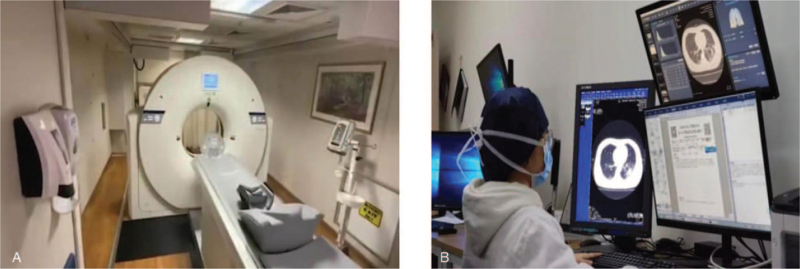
Vehicle-mounted CT machine and doctor remote operation scan. (A) Vehicle-mounted mobile CT (B) Doctors issue reports based on CT.
